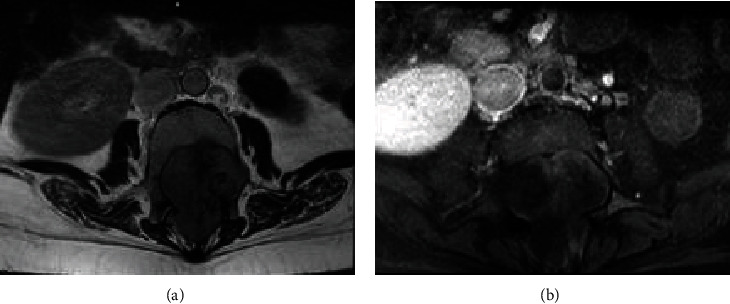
A lumbar spine MRI axial view ((a) T2 and (b) T1 with contrast).
